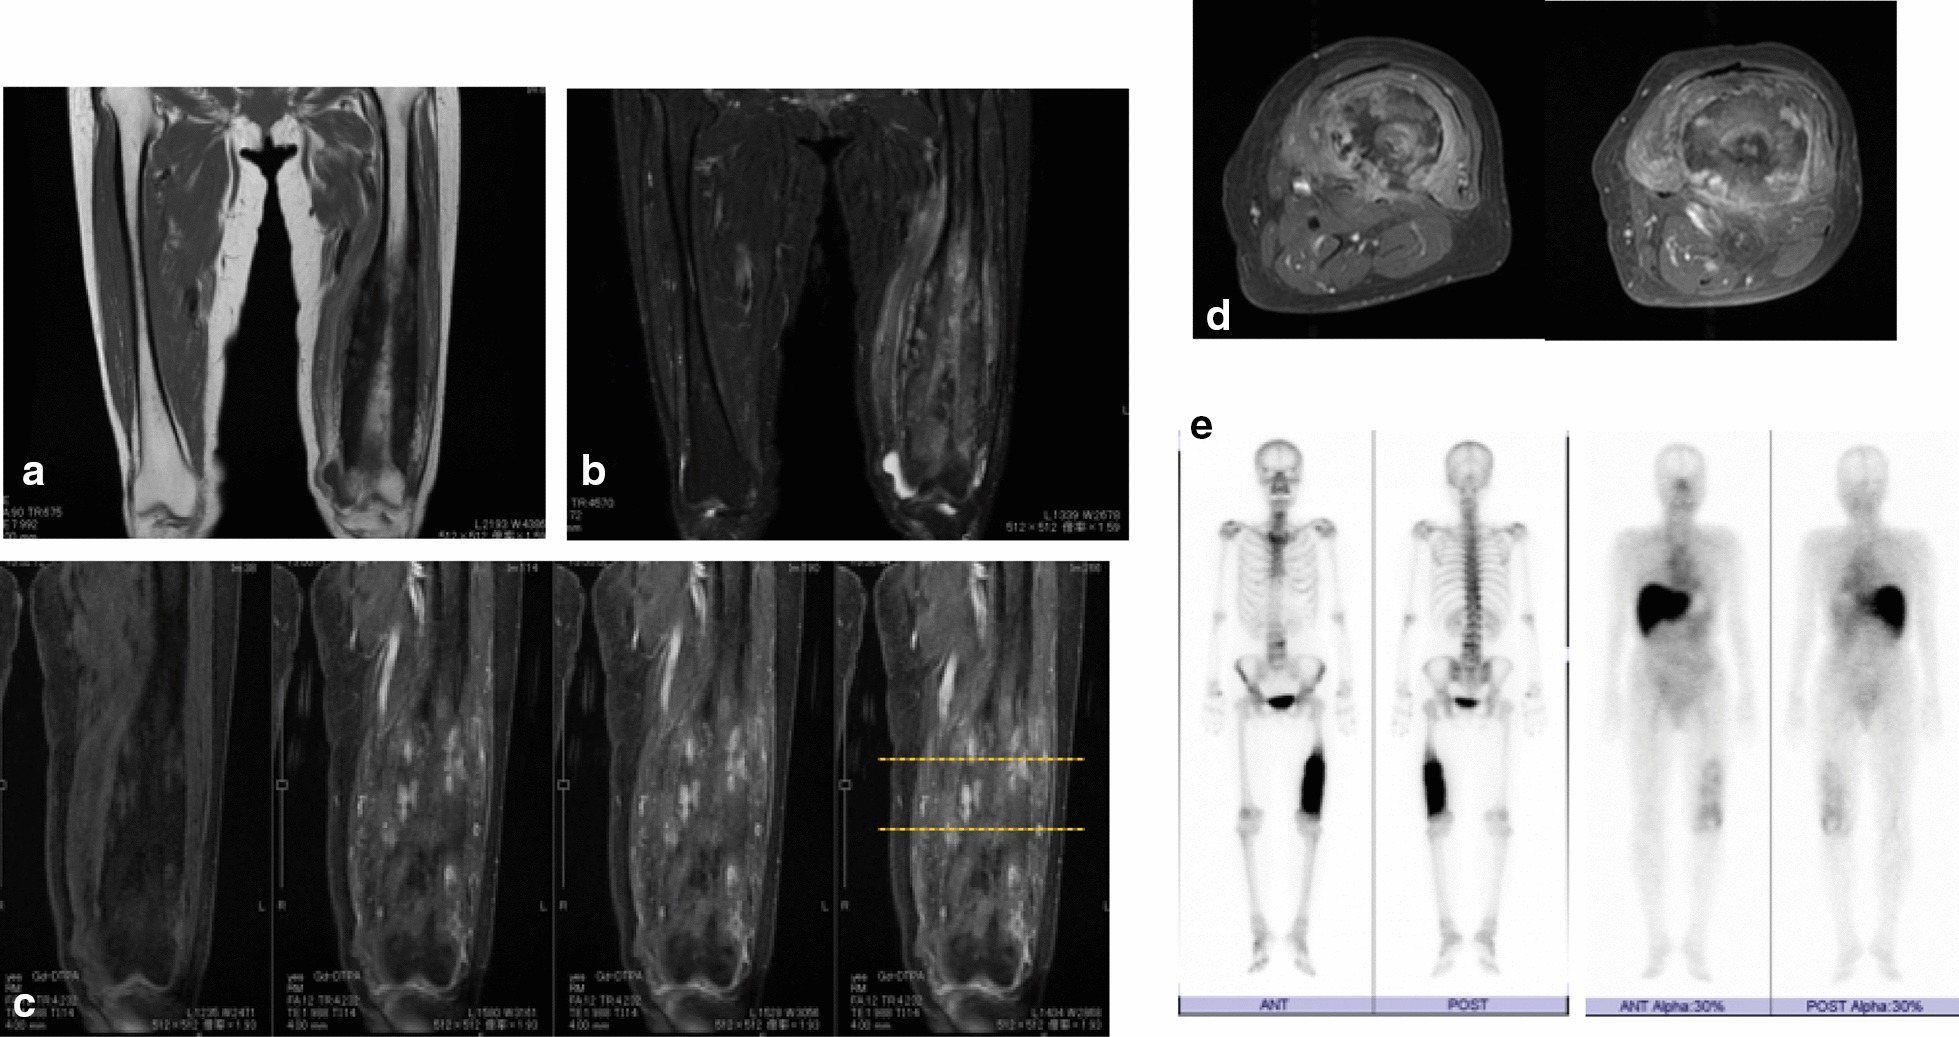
MRI and bone scan of the left femur. MRI image of the left femur. a T1-weighted image showing an extremely low signal intensity and a heterogeneous lesion in the sclerotic lesion and medullary cavity, respectively. The medullary cavity is narrower than that in the contralateral femur. b T2 fat-suppressed image showing heterogeneous signals in the sclerotic lesion and medullary cavity. c, d Gadolinium contrast MRI images showing partially high signals around the bone and axial image showing heterogeneous signals in the medullary cavity and soft tissue. e Bone scans showing significant uptake in the left femur
